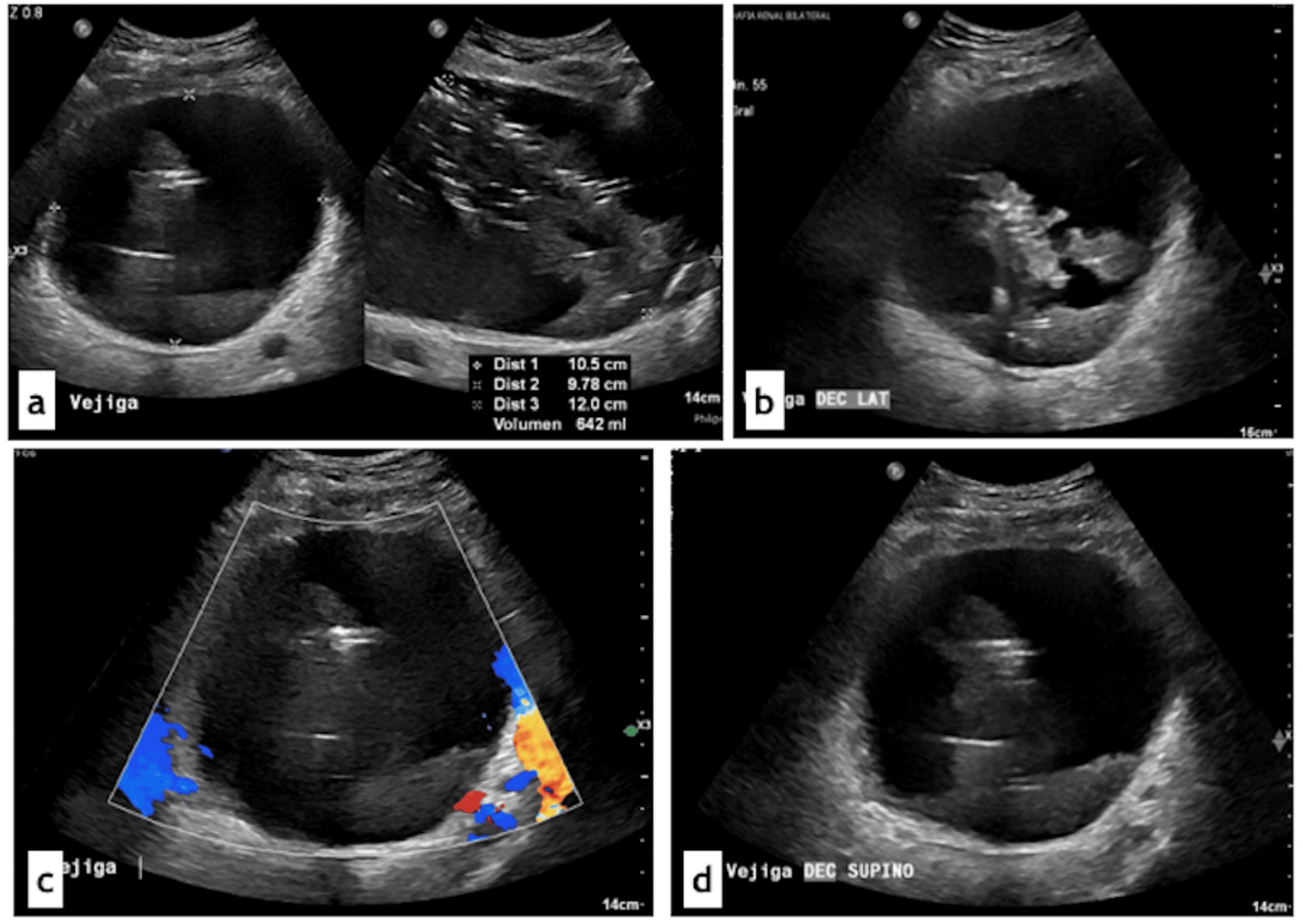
Bladder ultrasound. Bladder ultrasound demonstrated a bladder with an approximate volume of 642 cc. Inside, an irregular, mobile, hyperechoic features were identified, which changed position with patient movement. Multiple echogenic foci produced reverberation artifacts consistent with air locules. No ureteral jets were visualized. (a) Axial and sagittal bladder views showing an intravesical mass. (b) Axial view of the bladder depicting a mobile intravesical mass. (c) Image showing the absence of ureteral jets. (d) Axial bladder view showing sediment.
